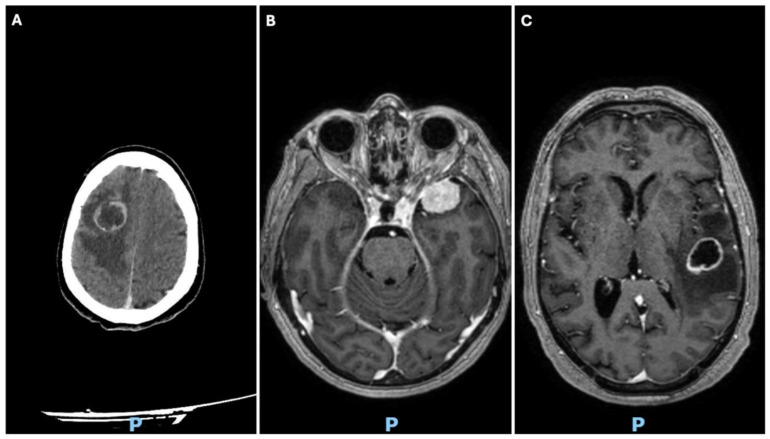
(A). Brain CT scan showing right frontoparietal necrotic lesion with ring-shaped contrast enhancement and perilesional edema. (B). T1-weighted MRI images showing the presence of a lesion with uneven intensity in the left sphenoid wing region. (C). T1-weighted MRI of the brain showing a left temporo-parietal lesion with ring-shaped hyperintensity and perilesional edema.
